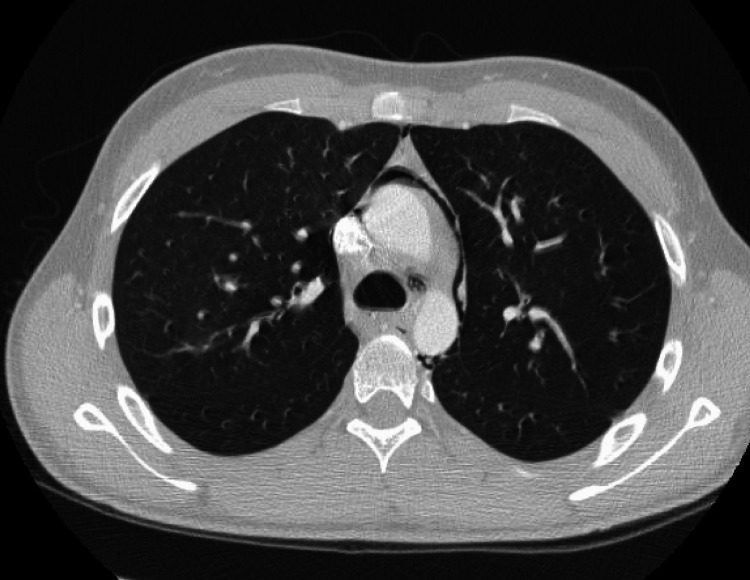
Computed tomography (CT) of the thorax pneumomediastinum extending to the cervical soft tissues.
